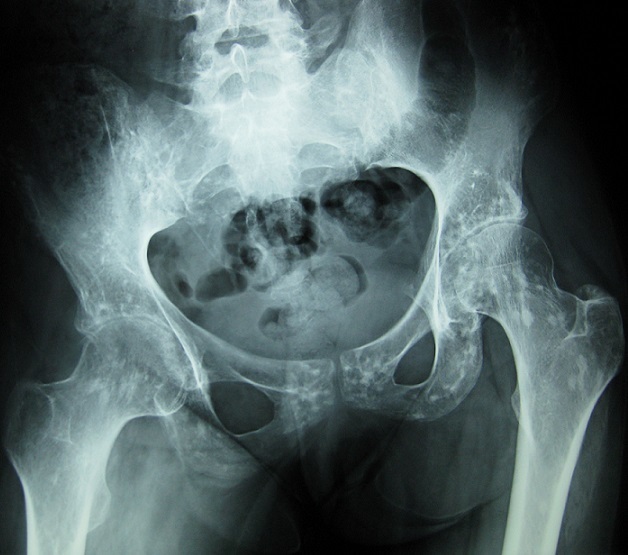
Radiographie standard du bassin révélant de multiples lésions condensantes arrondies ou ovoïdes symétriques siégeant sur les extrémités supérieures des fémurs, des cotyles, des branches ischio-pubiennes, de la symphyse pubienne, des ailes iliaques et du sacrum en rapport avec une ostéopoécilie associées à un pincement coxo-fémoral bilatéral avec érosions de la tête fémorale et du cotyle en rapport avec une coxite
